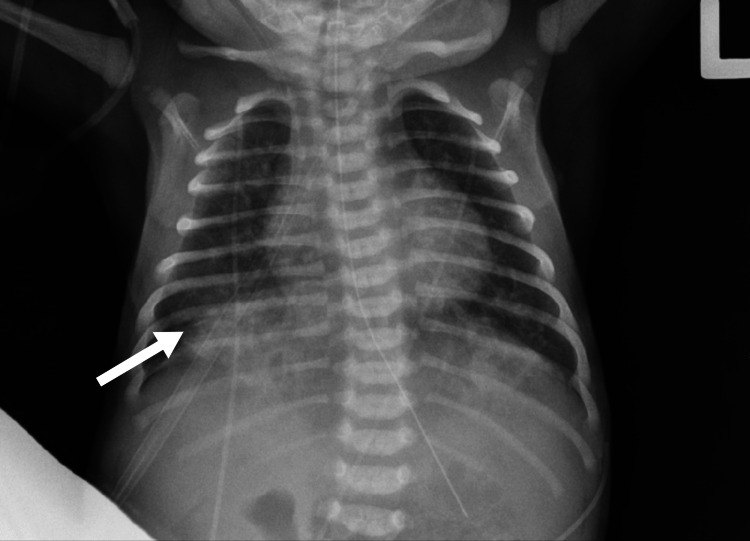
Chest X-ray on day of life 3: right lung base infiltrates increased (white arrow)
